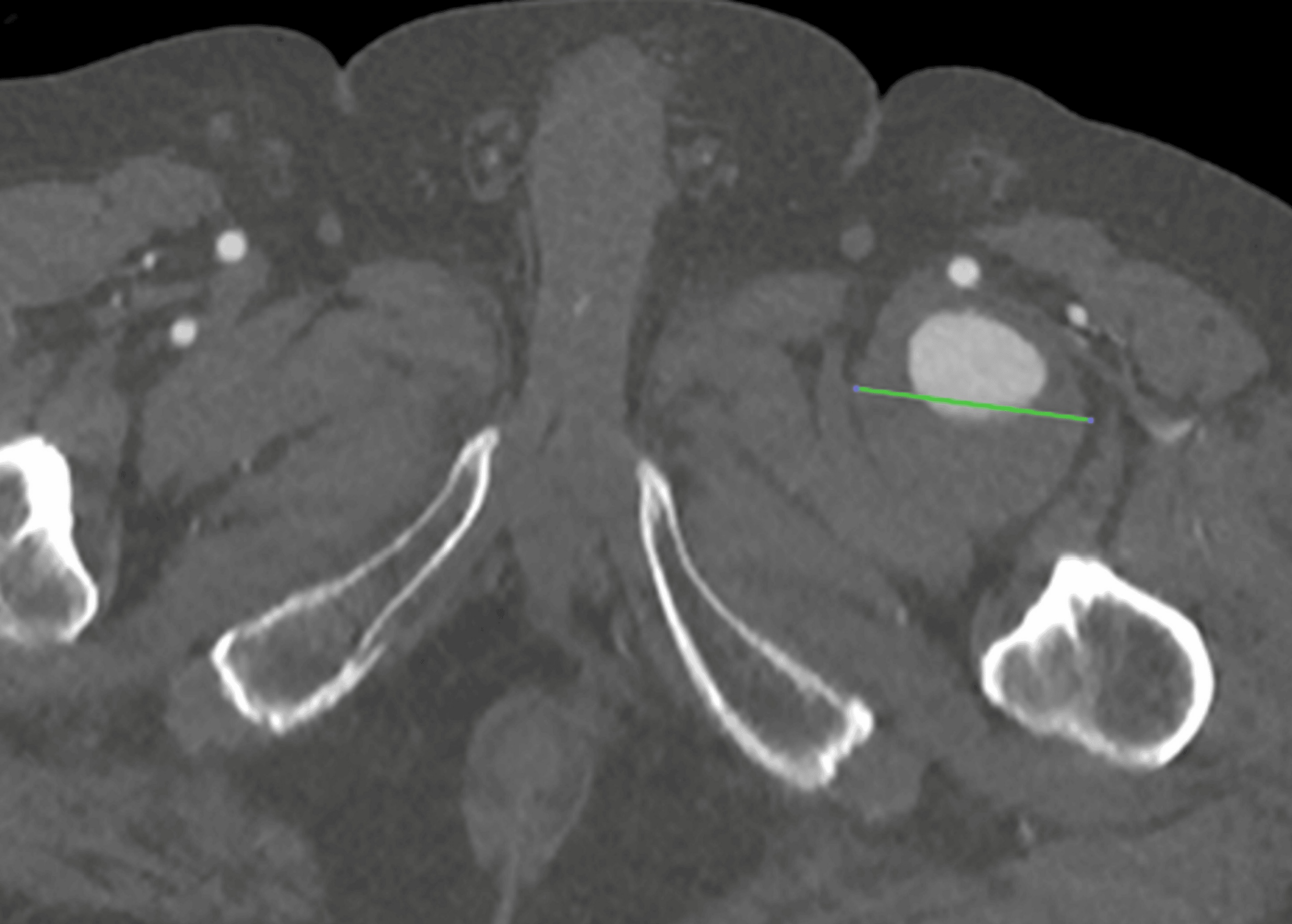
CT angiography, transverse plane It shows the left deep femoral artery aneurysm in the proximal third part of the vessel (transverse size: 5.1 cm - green line) with mural thrombus.
